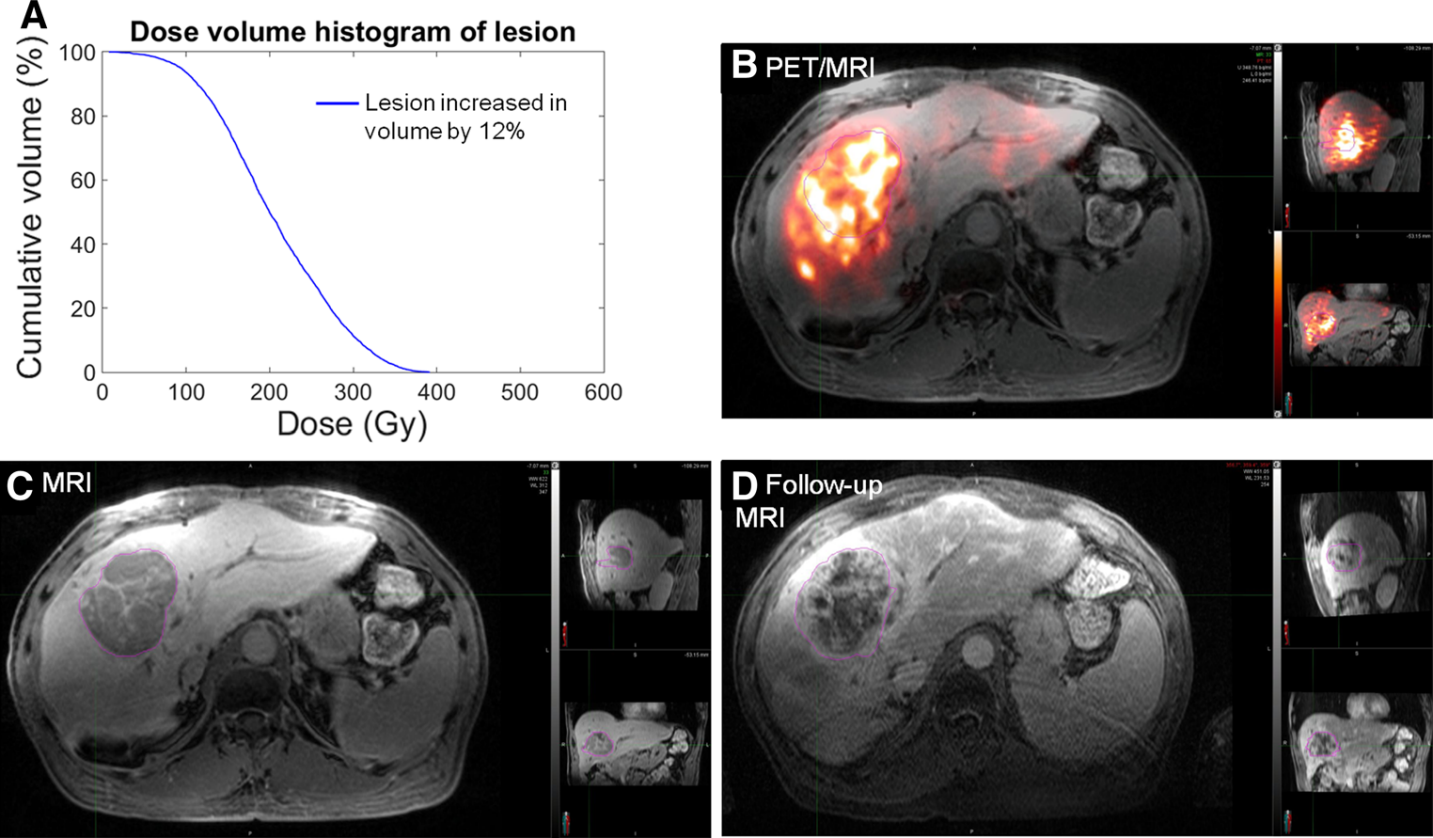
Hepatocellular carcinoma (HCC) lesion representing one of the three outliers among the hypervascular lesions (see Fig. 4). Despite a relatively high delivered dose (A), this lesion did not respond to therapy. PET/MRI (B) shows expected deposition. Baseline MRI (C) and follow-up MRI (D) show stable/no response as defined by RECIST/vRECIST
